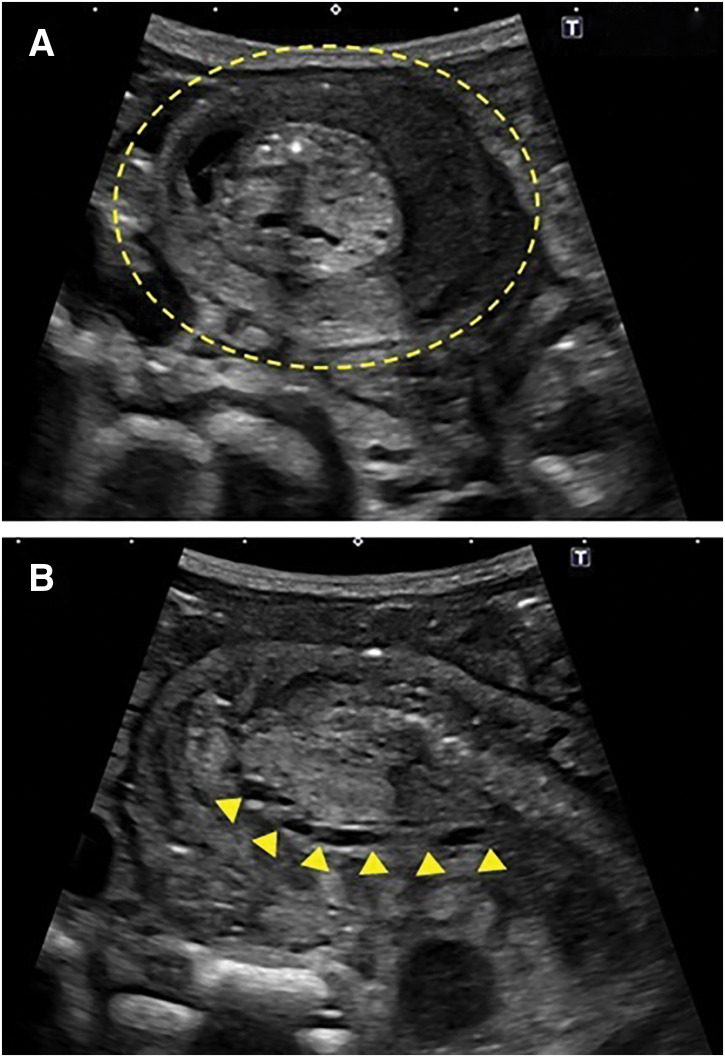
Sonography showing the small bowel intussusception. (A) Sonography showing the target sign in the right lower abdomen (dashed circle). (B) The nasojejunal tube passes through the intussuscepted bowel loops (arrowheads).
